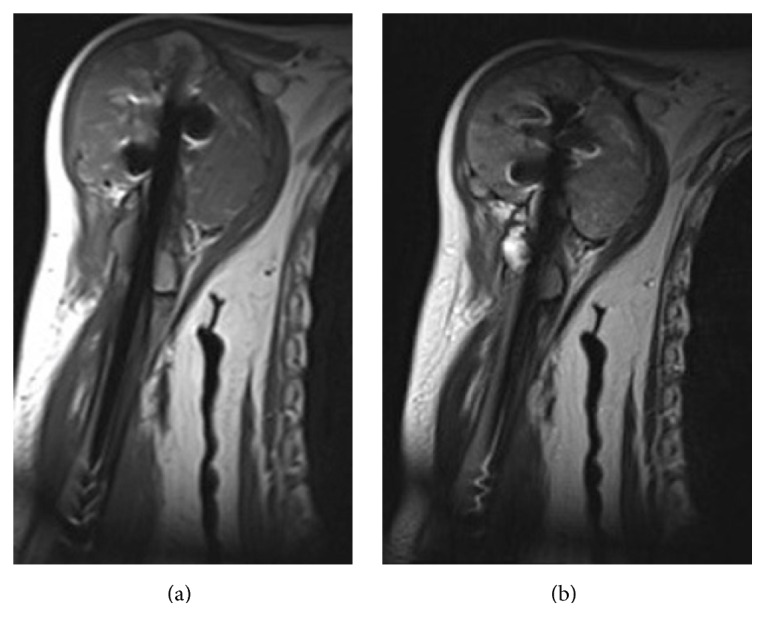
Magnetic resonance imaging (MRI) findings of the right humerus. (a) T1 and (b) T2 weighted imaging show mild high signal changes in the tumor lesion that expanded around the humeral nail.
